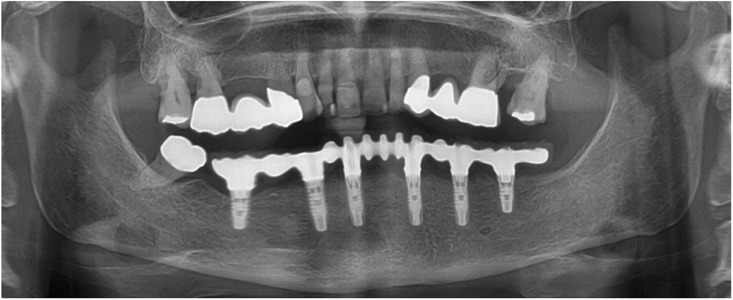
Panoramic radiograph at delivery of the final CAD/CAM FDP
